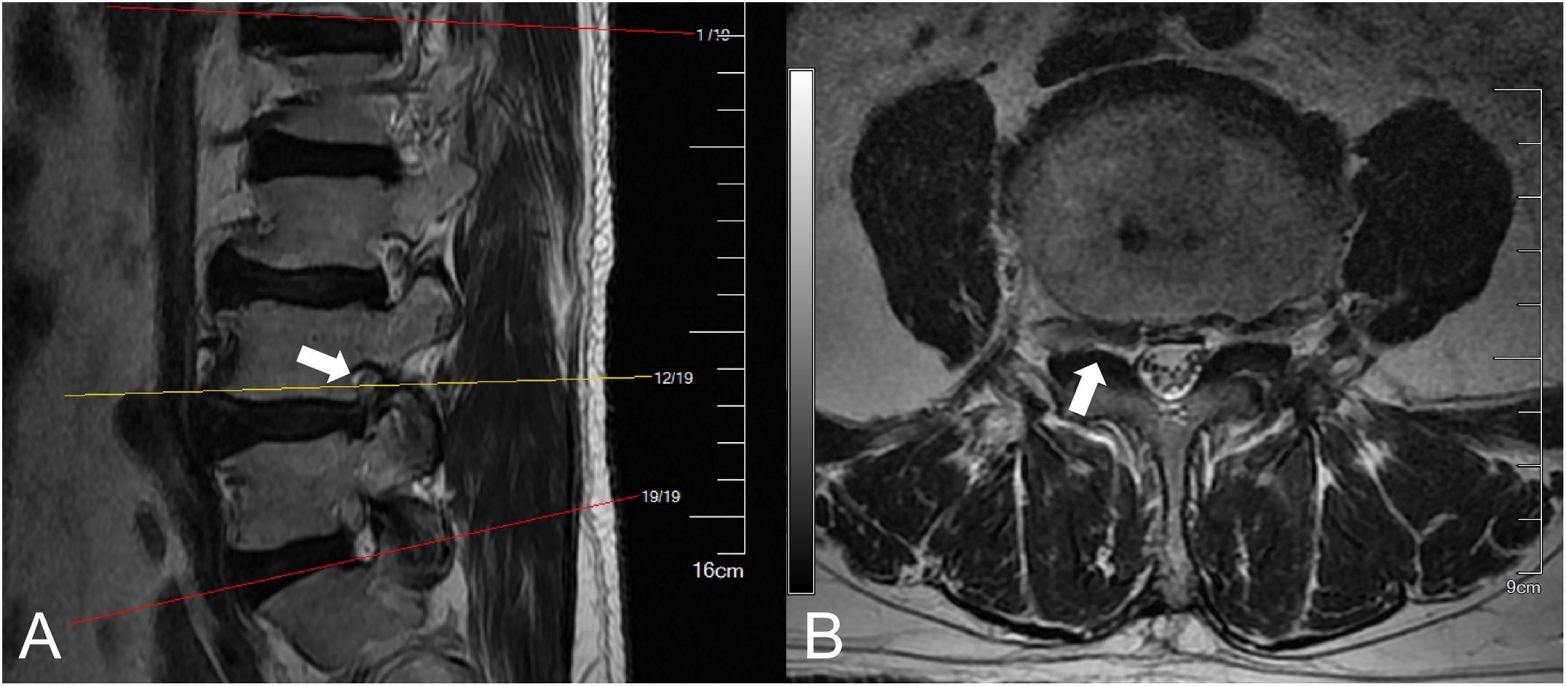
MRI views of the right L4/5 neural foramen. (A) Sagittal and (B) axial images demonstrated moderate foraminal stenosis. The exiting L4 nerve root is partially surrounded by epidural fat (white arrows), and there is no observable deformation of the nerve root.
